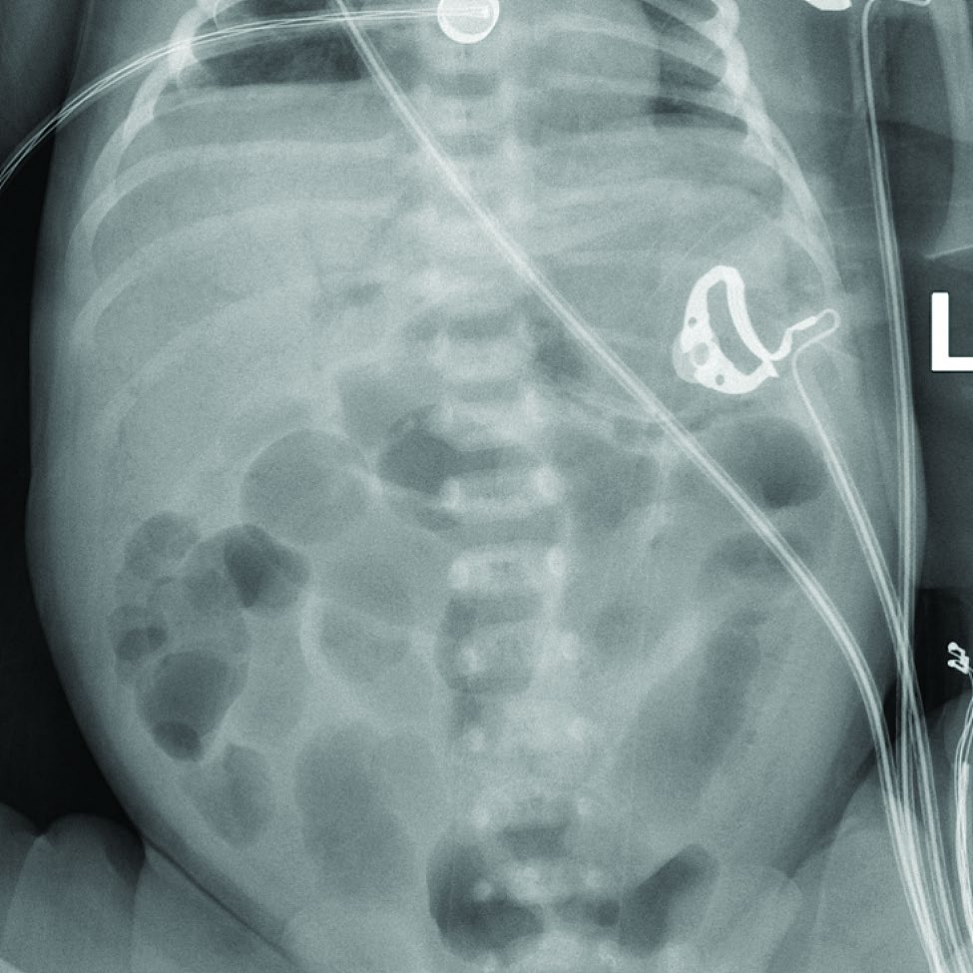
Plain abdominal X-ray (a) showed portal venous gas, bowel distension, widened inter-loop spaces, and focal pneumatosis intestinalis, without any free subdiaphragmatic air (pneumoperitoneum)
